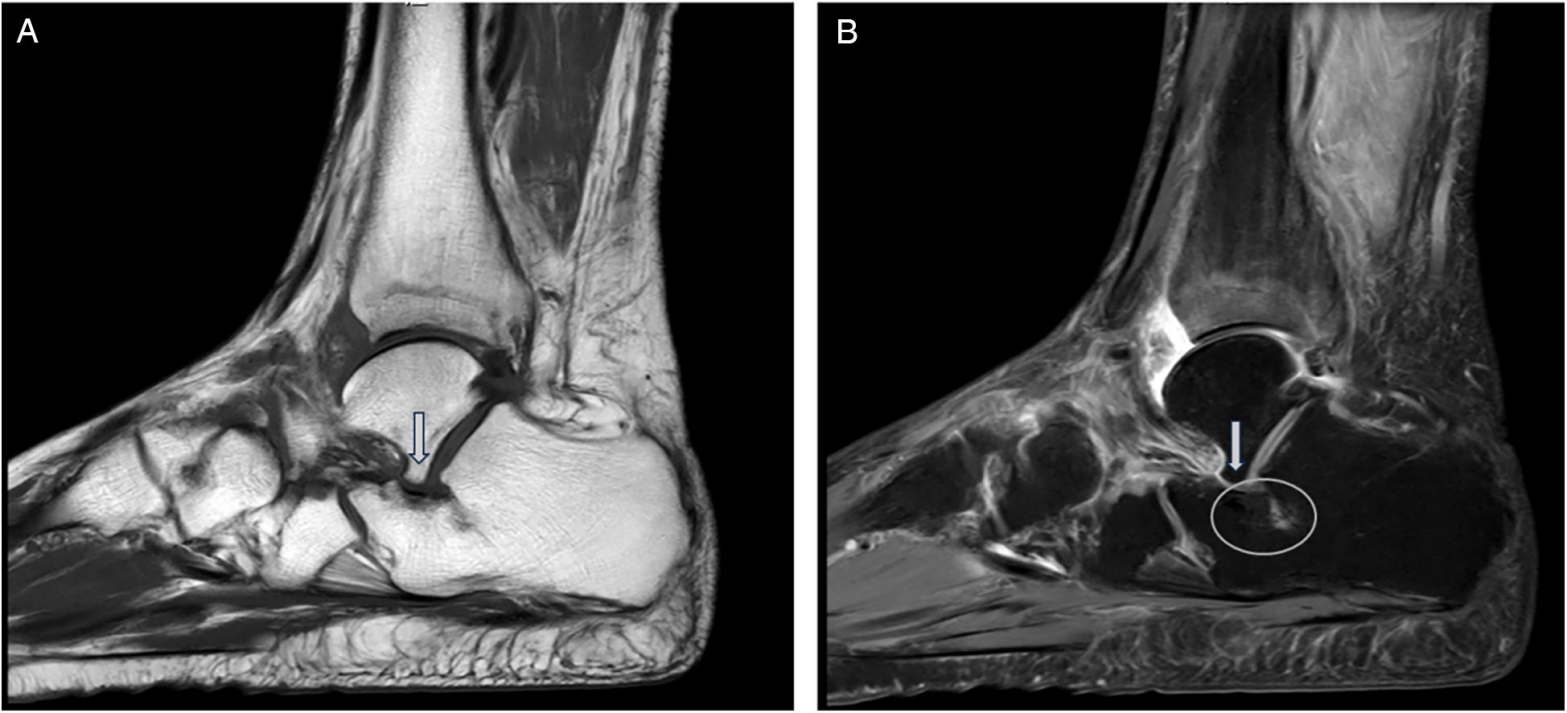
AALTF is anatomically identified as a bony protuberance arising from the lateral talar process and extending anteriorly to articulate with the calcaneus. (A) It is typically observed on sagittal T1-weighted MRI as a discrete osseous structure that contributes to talocalcaneal impingement in patients with flatfoot deformities (arrow). (B) Sagittal MRI (T2) imaging proving the presence of an osseous protuberance and the bone marrow edema located at the opposing calcaneal side of the AALTF (circle).
